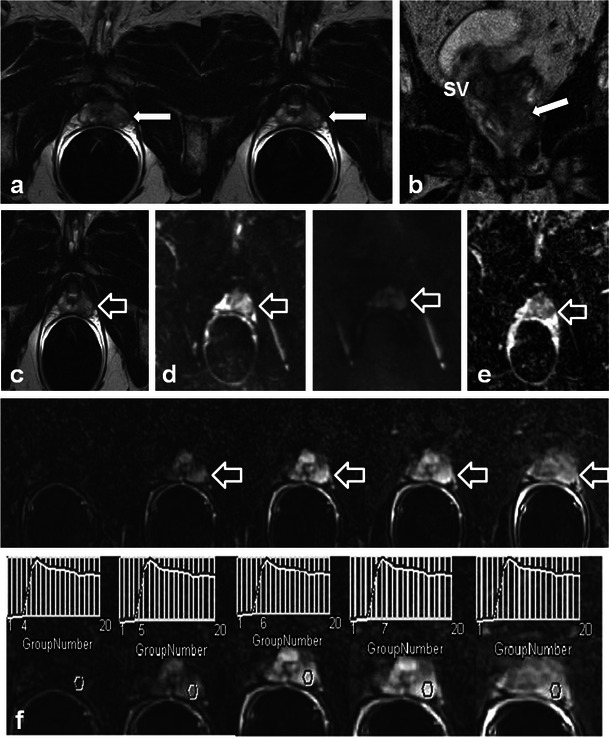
A 68-year-old man with prior external RT and biochemical failure with subsequent transrectal ultrasound guided biopsy demonstrating Gleason score 8 recurrent tumour in the left mid and basal peripheral zone. Multi-parametric endorectal coil prostate MRI depicts atrophic prostate gland and seminal vesicles with low T2 signal intensity and poor delineation of prostate zonal anatomy on axial and coronal T2 TSE images (a, b and c) which are typical post-RT changes. There is an intermediate T2 signal intensity area in the left mid and basal peripheral zone (*white arrows*), which is bulging the prostate capsule. Diffusion-weighted images (d) with B0 and B1,000 and ADC map e demonstrate restricted diffusion in this location (*open white arrows*). Corresponding axial pre- and selected post-dynamic-contrast-enhanced GRE images with semi-quantitative contrast curves demonstrate corresponding early rapid enhancement with steep upslope (*open white arrows* in f) and contrast washout in the area of known recurrent tumour
